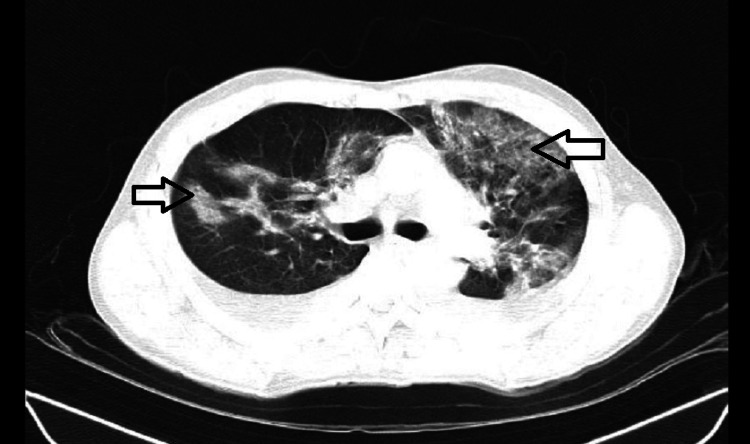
HRCT thorax revealing multifocal areas of ground glass appearance in both the upper lobe, left lingular lobe, and left lower lobe suggestive of possible pulmonary contusion HRCT: high-resolution computed tomography
